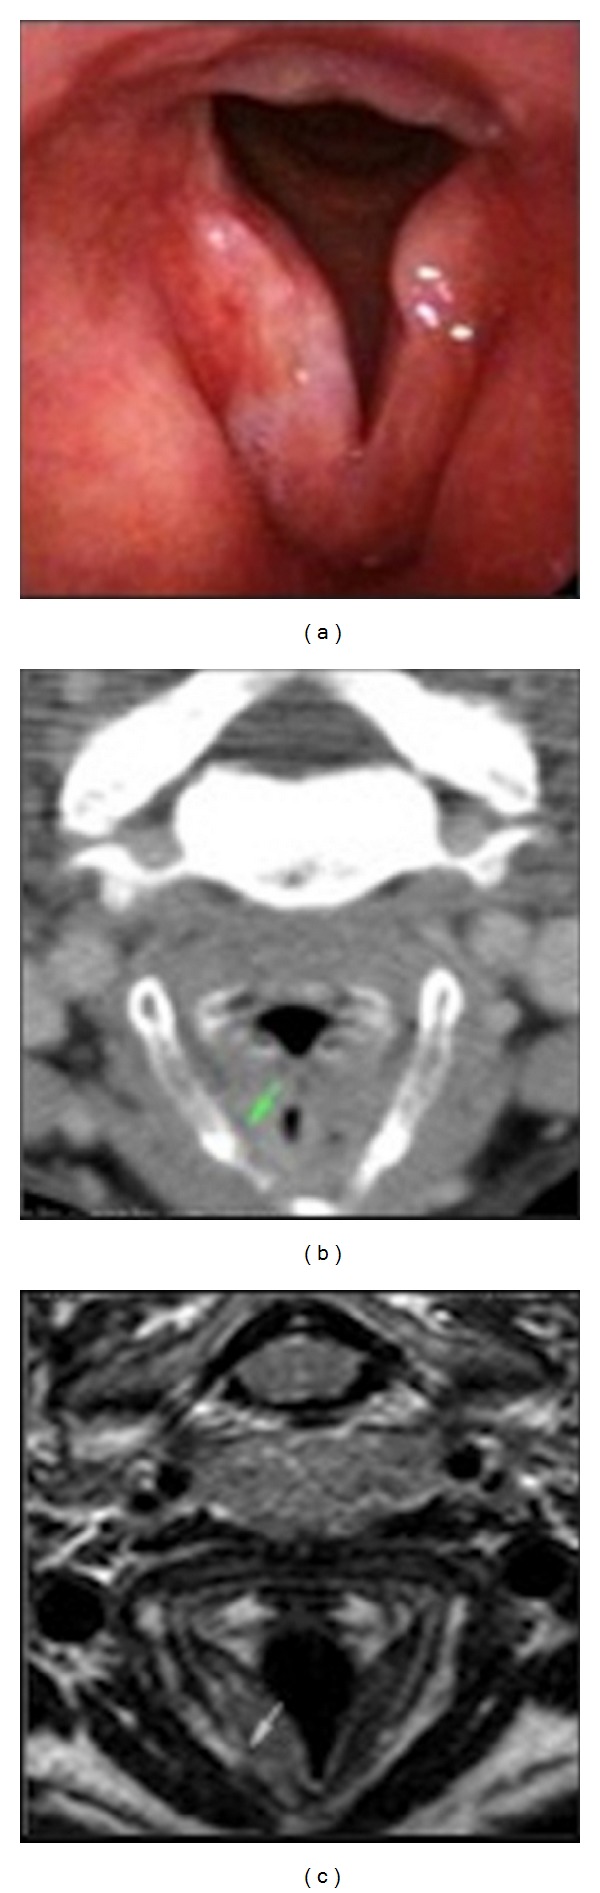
Carcinoma of the right vocal cord: (a) endoscopic view; (b) CT image: the paraglottic space seems preserved (green indicator) without cartilaginous alterations; (c) T2w MR image: the paraglottic space seems involved with focal invasion of the thyroid cartilage (green indicator).
